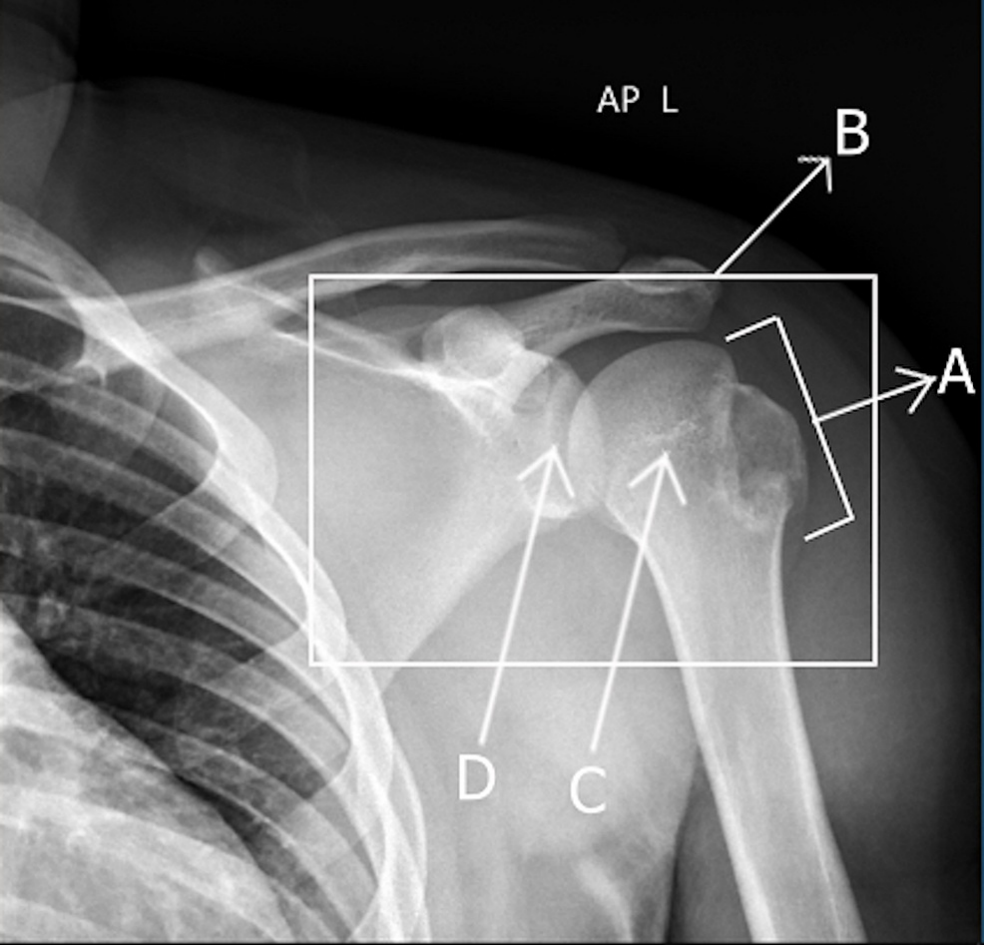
Anterior-posterior X-ray showing the left glenohumeral joint post-reduction with the avulsion fracture of the greater tuberosity still visible. The avulsion fracture of the greater tuberosity is still visible (A), and the left glenohumeral joint post-reduction (B) can be seen. The humeral head (C) is back in the socket articulating with the glenoid (D).
